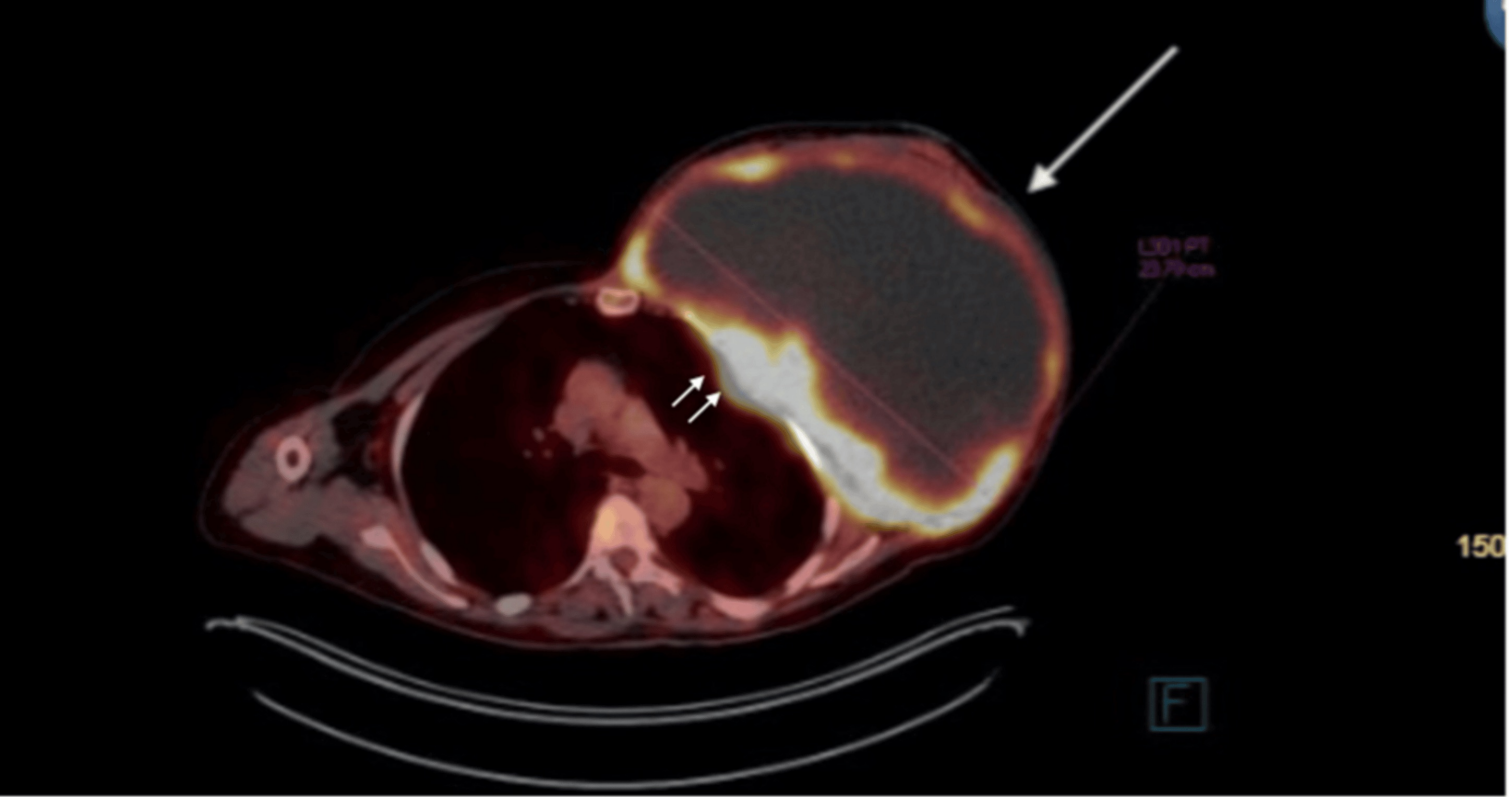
Huge left breast mass observed on the PET-CT with high peripheral FDG uptake The single arrow points at the lesion, and the double arrows point at the attachment and invasion to the chest wall. FDF: Fludeoxyglucose-18
